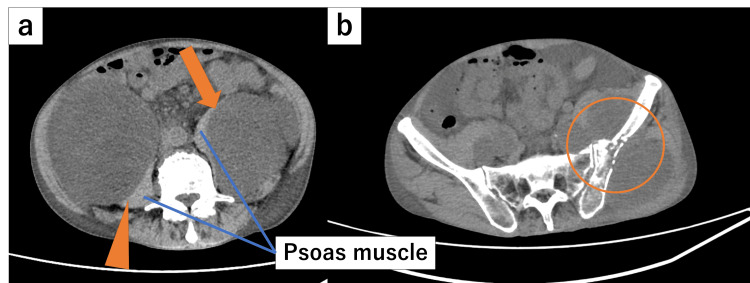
Chest and abdominal computed tomography in the axial plane before radiation therapy. (a) A large disseminated lesion infiltrating the left psoas muscle was identified as the cause of malignant psoas syndrome (arrow). Another disseminated lesion infiltrated the right psoas muscle (arrowhead), but there were no symptoms on the right side. (b) A separate disseminated lesion infiltrating the left iliac bone. The patient experienced left buttock pain (circle).
